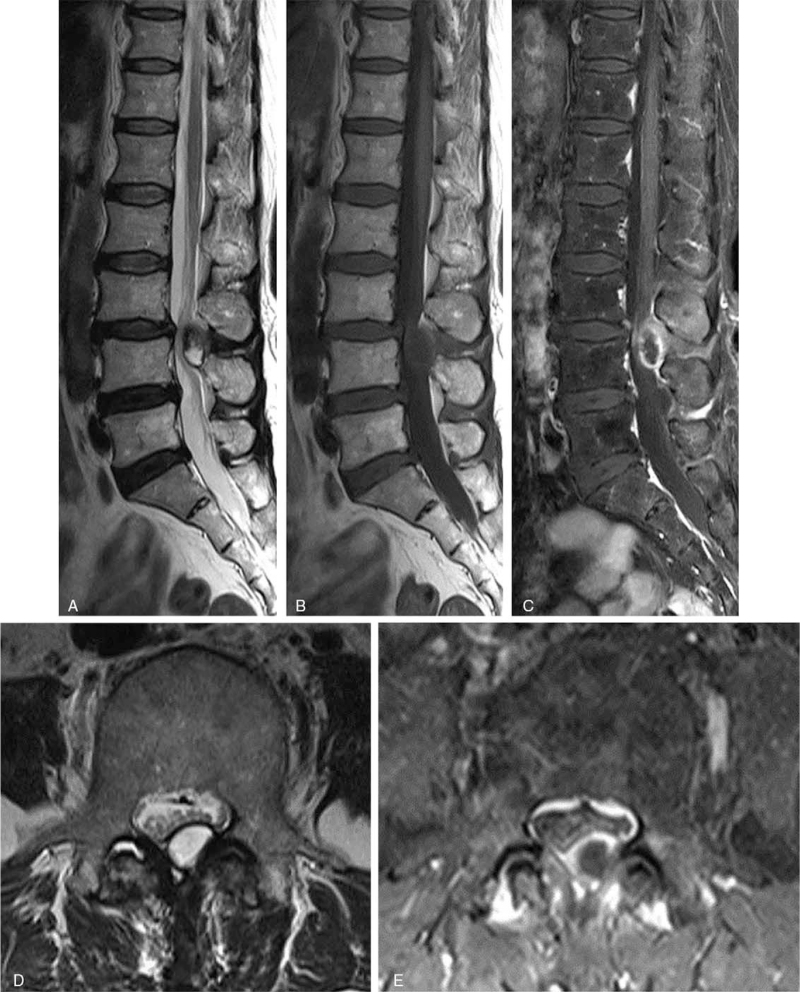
Preoperative lumbar MRI without contrast enhancement demonstrated a posterior epidural lesion at the L3-4 level with thecal sac compression (A, B, and D). After contrast enhancement, this mass-like lesion showed heterogenous signal intensity and peripheral rim enhancement (C and E).
